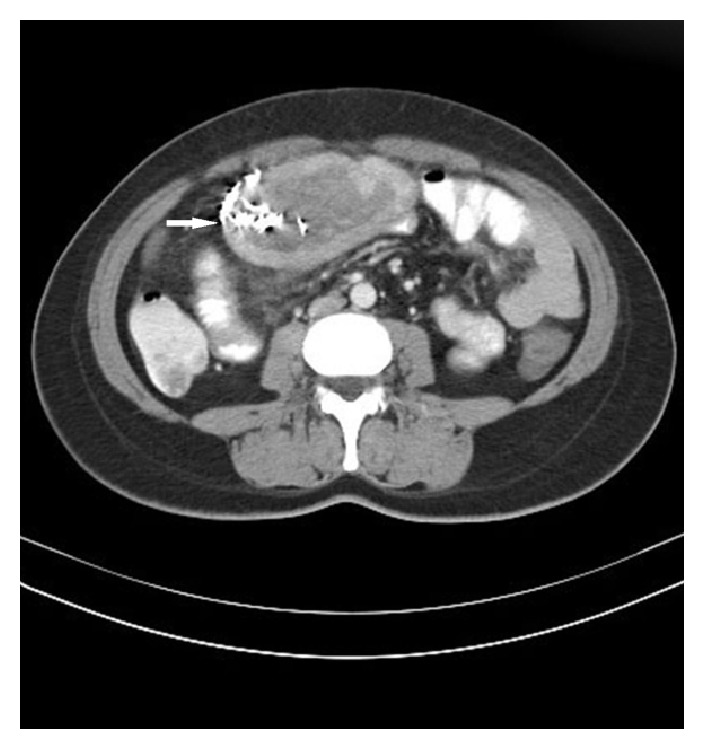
Gossypiboma in abdominal computed tomography.
